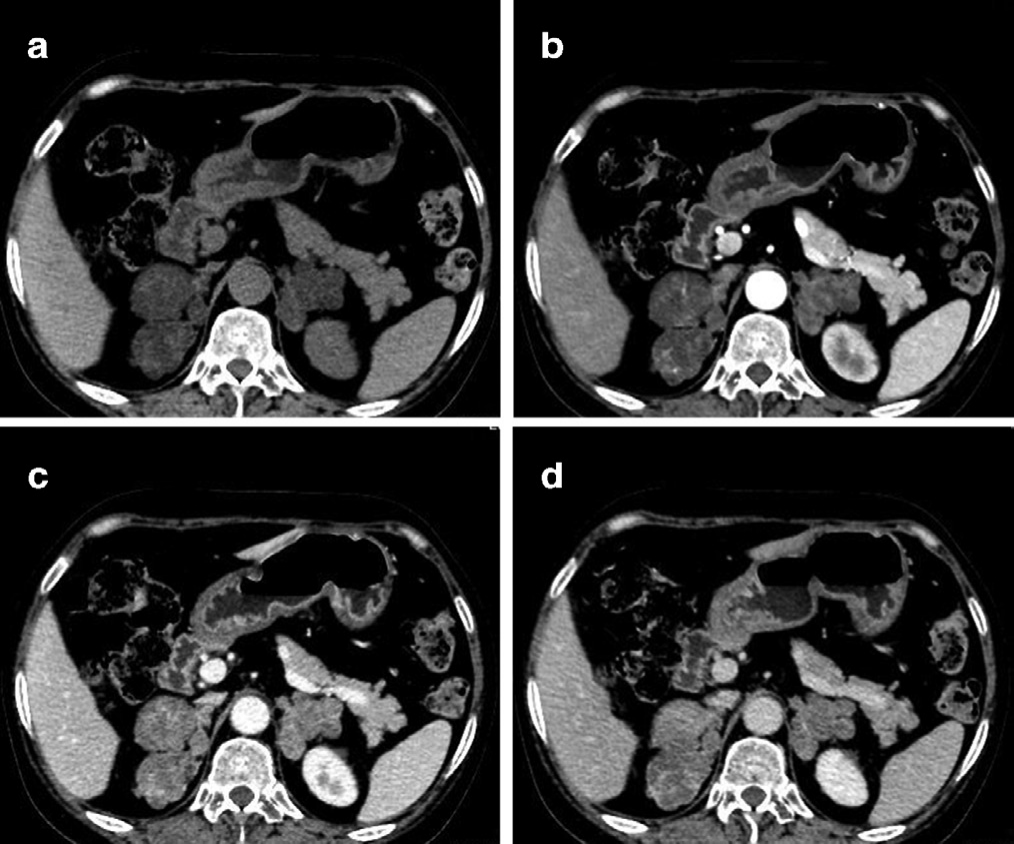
CT showing hypodense lobulated lesions on the right and left adrenal glands (A); Arterial phase images of enhanced CT revealing mild-to-moderate dotted and linear enhancement of the bilateral adrenal lesions (B); Bilateral adrenal lesions showing moderate enhancement in the portal phase (C); The degree of enhancement of the lesions in the venous phase decreased slightly (D) CT: computed tomography
